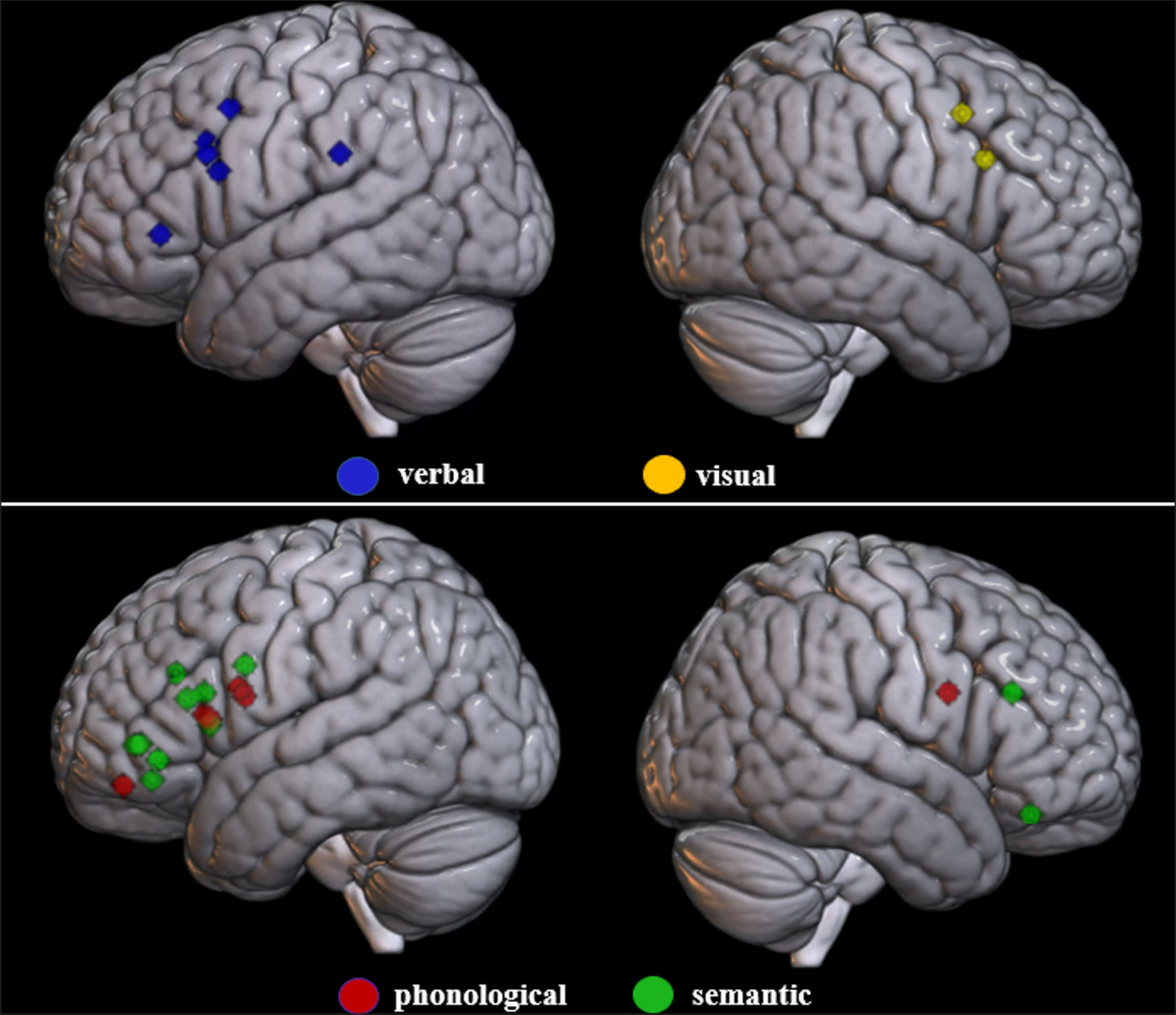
Summary of the activity peaks observed in the left and right frontal lobes for between-domain contrasts of RI. *Notes*: This figure was built by extracting first the MNI coordinates associated with contrasts between verbal semantic, verbal phonological and/or visual RI from the studies reported in this review. The selected MNI coordinates were then assembled using the WFU Pick Atlas (Maldjian et al., 2003, 2004) for each domain and displayed with a sphere shape of 5mm radius. These WFU-generated masks were then overlaid on a 3D render MRI template using MRICroGL (http://www.nitrc.org).
